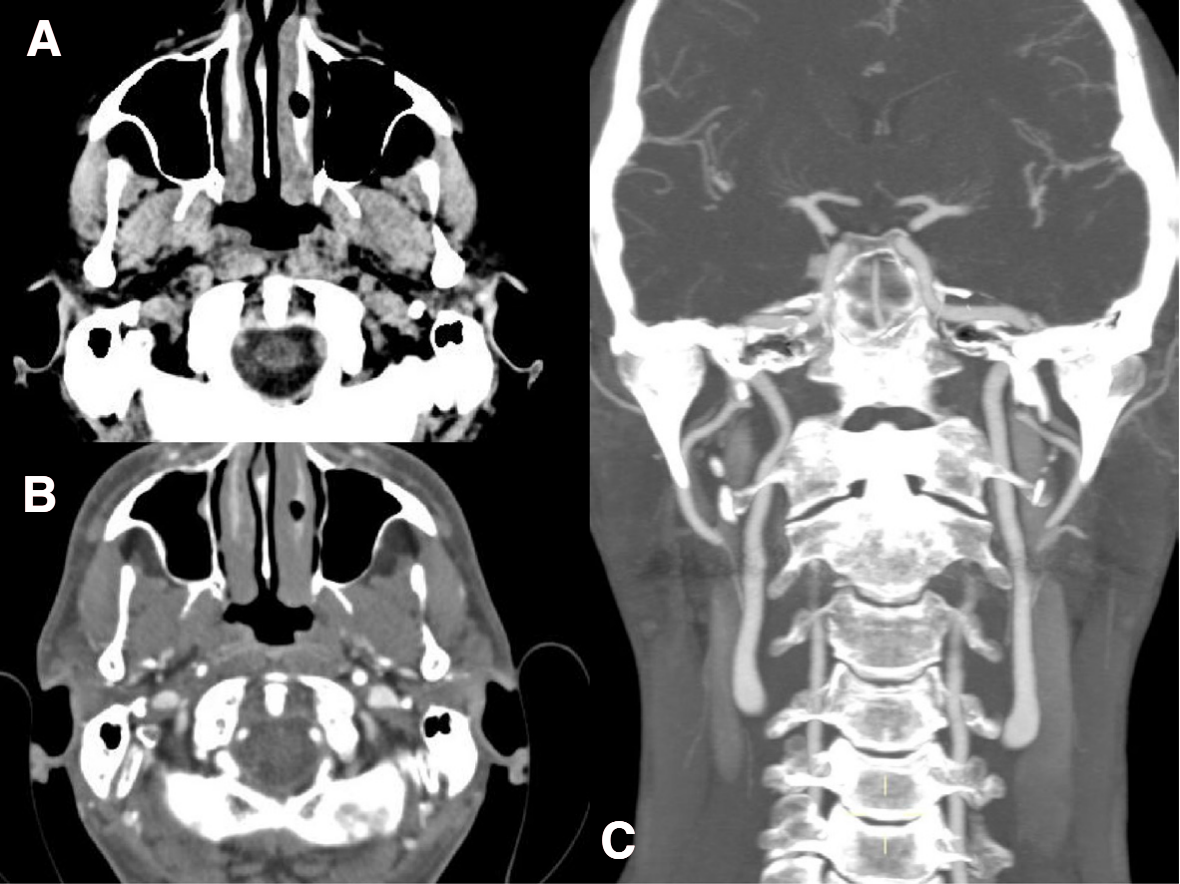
CT findings (performed in emergency department). A. Non-contrast axial CT shows normal findings. B. Axial CTA shows only a subtle asymmetry of the internal carotid lumen filling by contrast and was reported as negative for artery dissection. C. Coronal CTA shows normal findings.
